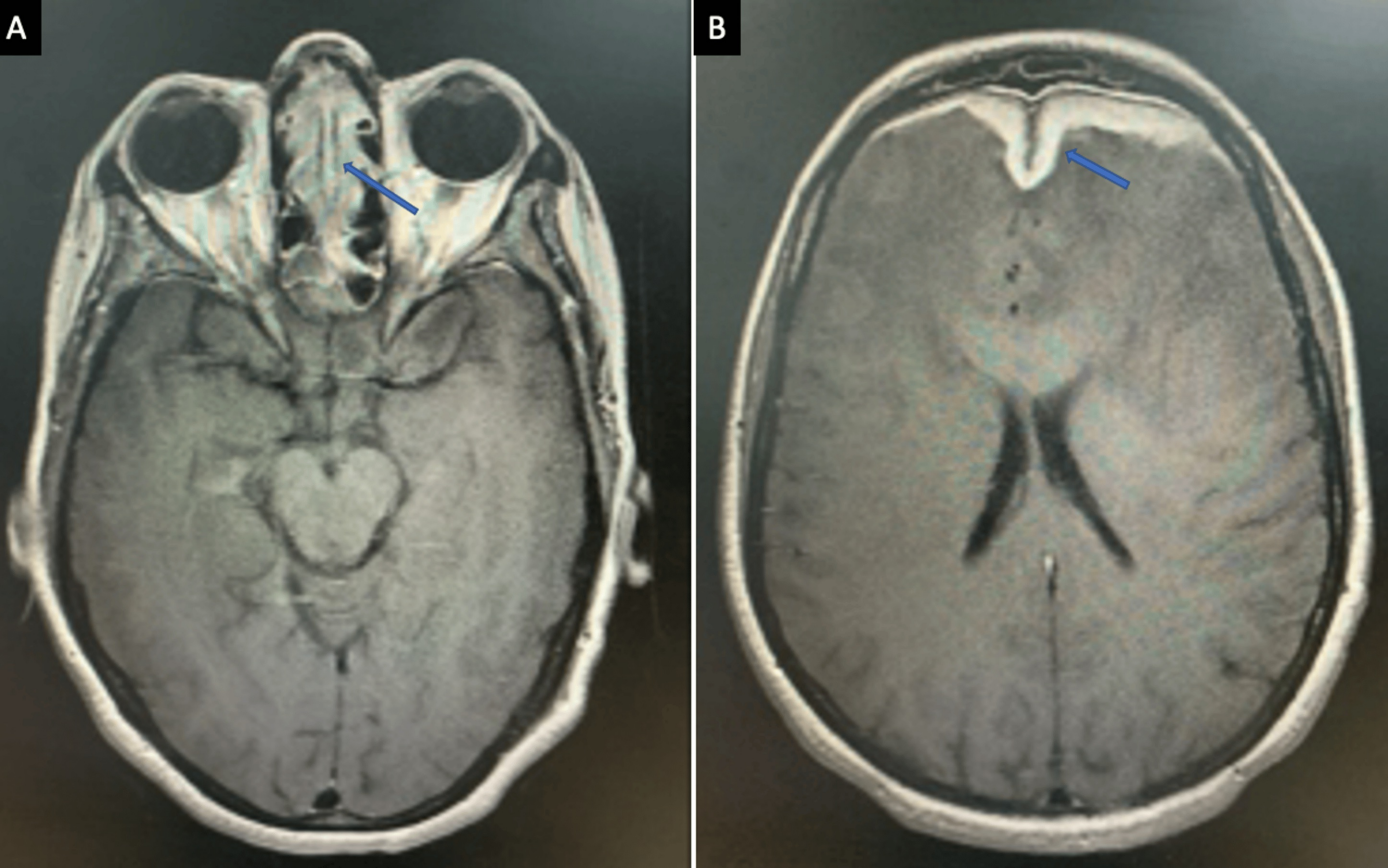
(A) A case of chronic invasive fungal infection displaying enhancing mucosal thickening in bilateral ethmoid sinuses (↗) (MRI, post-contrast T1 axial). (B) There is an intracranial extension of the disease via cribriform plate with pachymeningeal enhancement along the falx and dura of both frontal lobes (↗) (MRI, post-contrast T1 axial). MRI: magnetic resonance imaging
